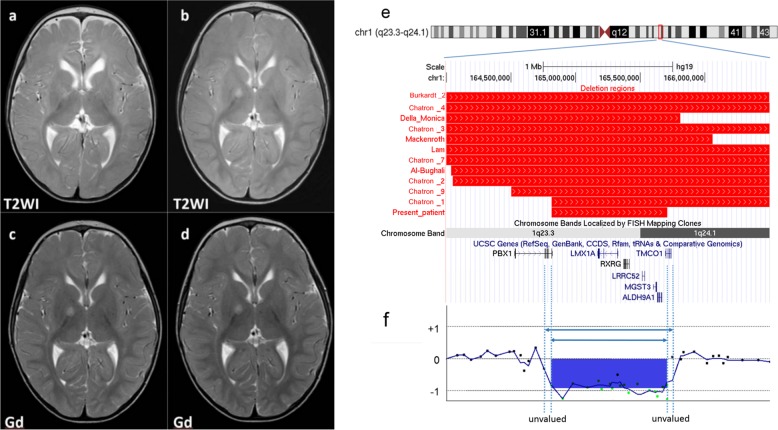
Results of brain magnetic resonance imaging and genome mapping around the 1q23.3q24.1 region. Axial T2-weighted images were taken at a, c 12 months and b, d 19 months. c, d A T2 high-intensity area found in the right genu of the internal capsule was not enhanced by gadolinium administration. e The deleted regions in our patient and in patients reported previously are shown by red bars. f Results of chromosomal microarray analysis of our patient
